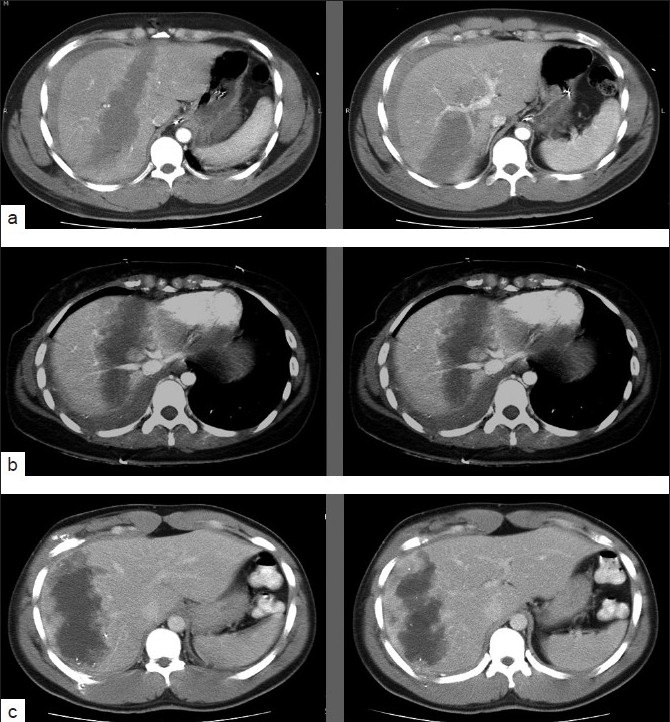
CT scans of the three patients with high-grade liver injuries treated nonoperatively (a) Patient 1, (b) Patient 2, (c) Patient 3
